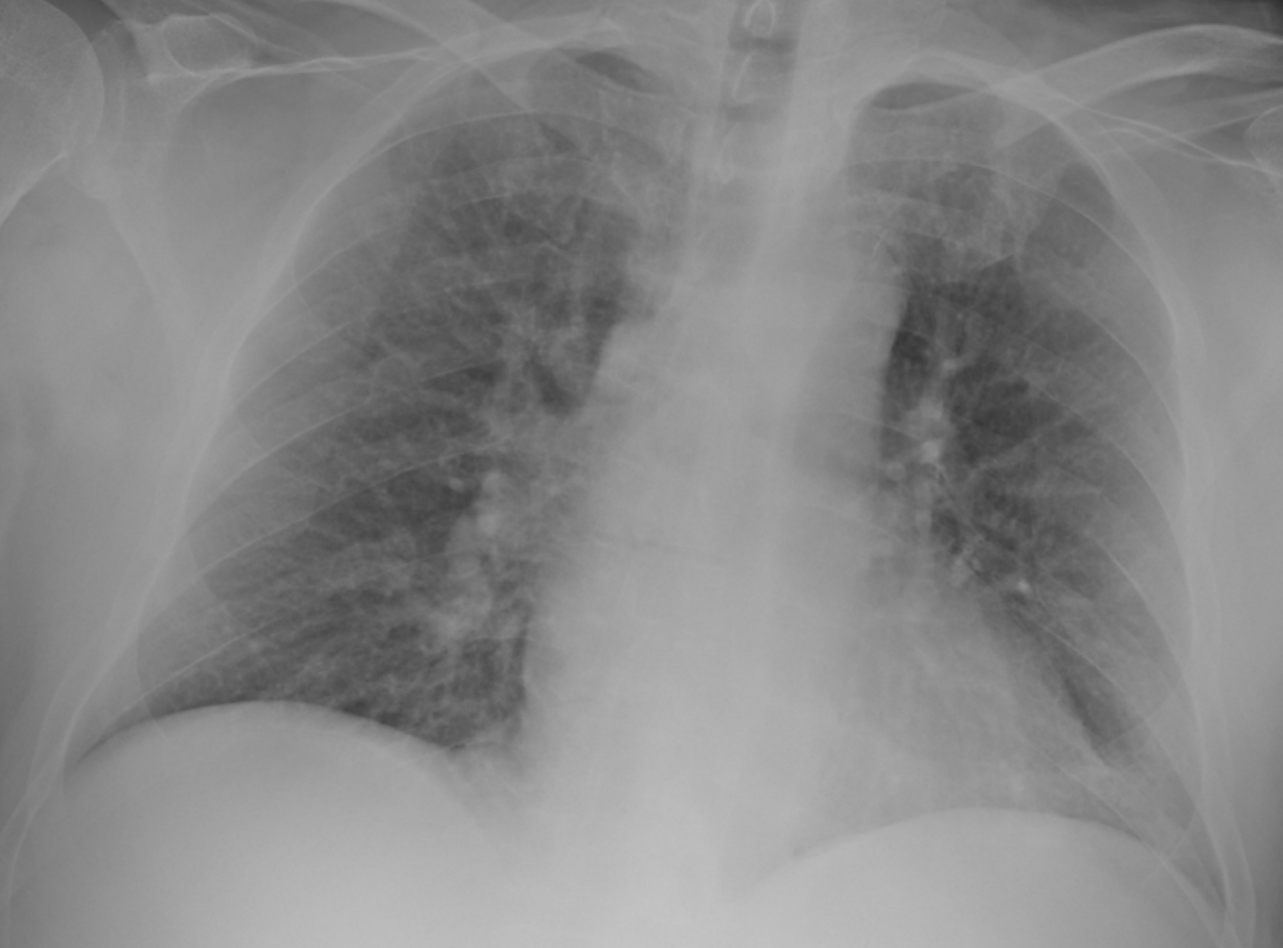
X-ray of the Chest (coronal view)
